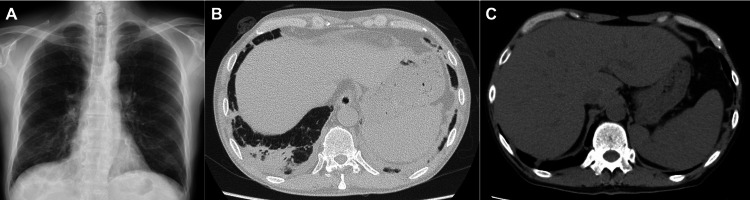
Findings at Initial Presentation (A) Chest radiograph shows no obvious abnormalities. (B) Chest CT demonstrates consolidation in the right lower lobe adjacent to the pleura. (C) Abdominal CT reveals hepatosplenomegaly without gallbladder enlargement or wall thickening.
